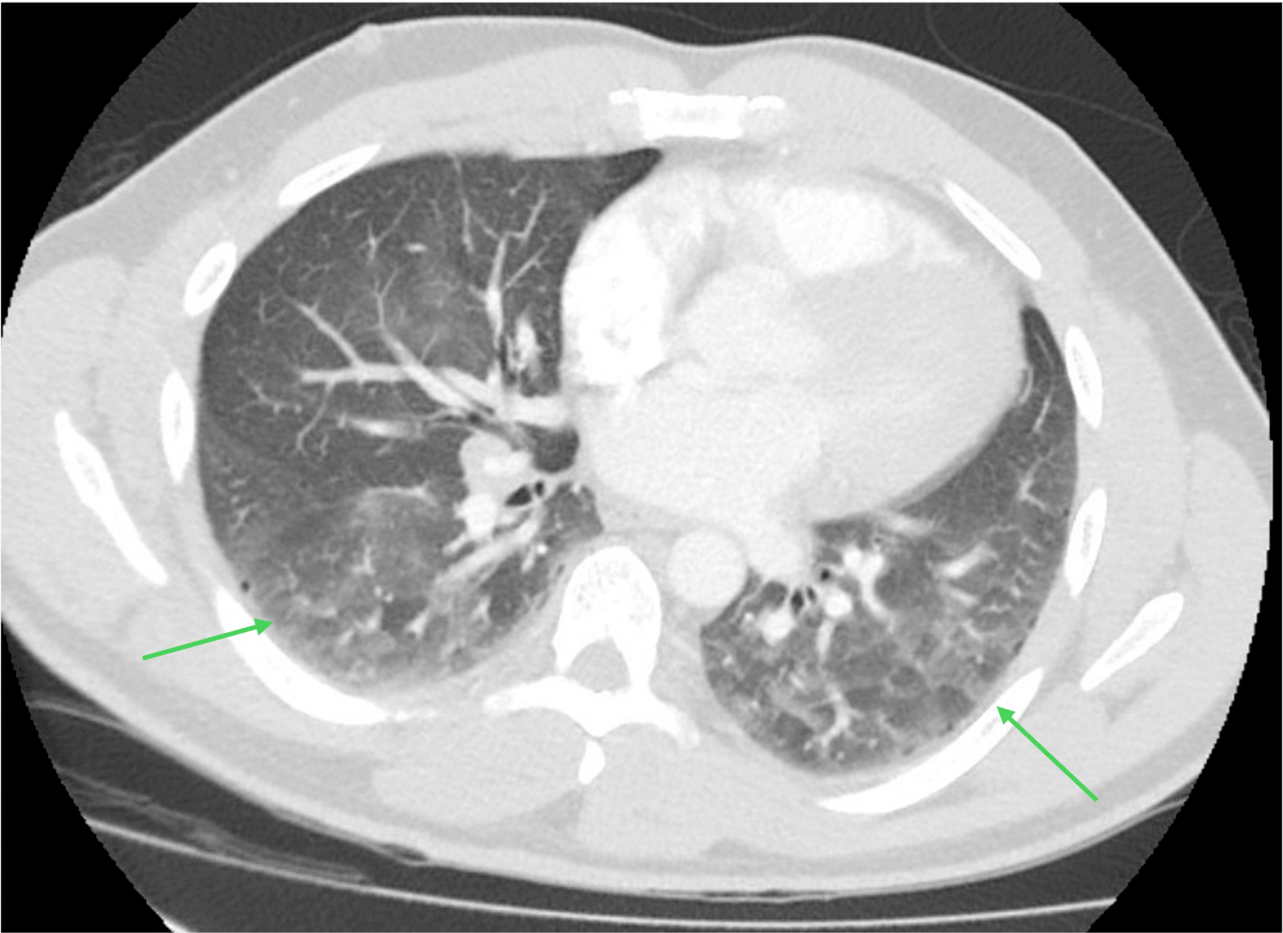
CTA showing ground-glass opacities in the lower lobes CTA, computerized tomography angiogram.
